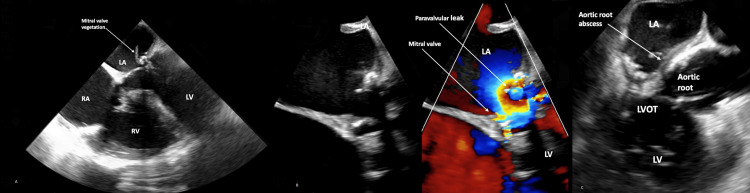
Transesophageal echocardiogram A. Mid-esophageal four-chamber view demonstrating a mobile, stalk-like filamentous vegetation attached to the mitral annuloplasty ring. B.  Mid-esophageal view of the mitral valve demonstrating a paravalvular leak. C. Transesophageal echocardiogram: mid-esophageal aortic valve long axis view demonstrating echolucency suspicious for aortic root abscess.
